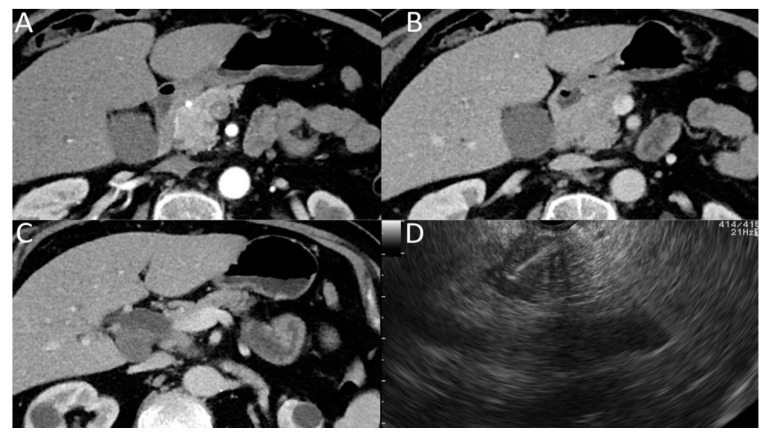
Isoattenuating pancreatic carcinoma on CT in pancreatic (A) and portal venous phase (B) with dilated common bile duct (C). EUS-FNA confirming the tumor of the head of the pancreas (D).
